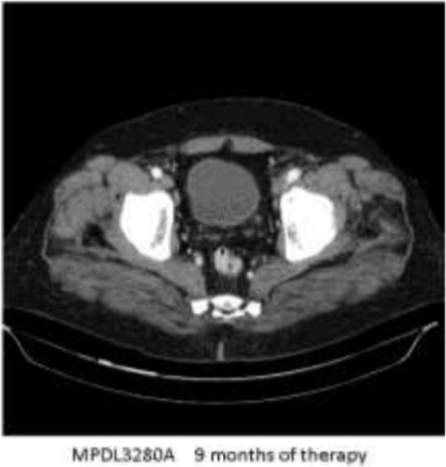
Bladder CT - 9 Months of Therapy. Complete Response
